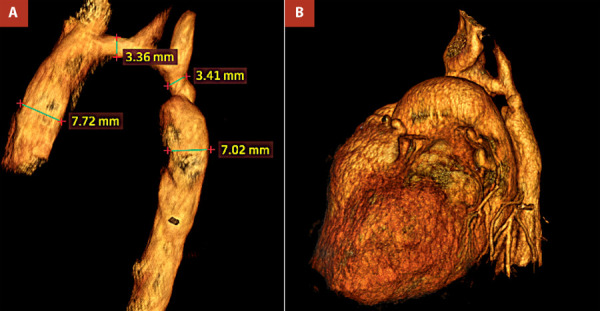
A. Three-dimensional volume-rendered computed tomography (CT) reconstruction of the thoracic great vessels demonstrates severe hypoplasia of the aortic arch, with significant narrowing of the proximal and distal segments measuring 3.36 mm and 3.41 mm, respectively. A markedly enlarged patent ductus arteriosus (PDA), measuring 7.02 mm, connects the pulmonary artery to the descending aorta. Postductal dilation of 7.72 mm is observed, consistent with retrograde systemic perfusion via the PDA. B. The 3D volume-rendered CT angiogram reveals a markedly hypoplastic transverse aortic arch, with critical narrowing at the level of the PDA, severely compromising the continuity of systemic flow through the arch.
